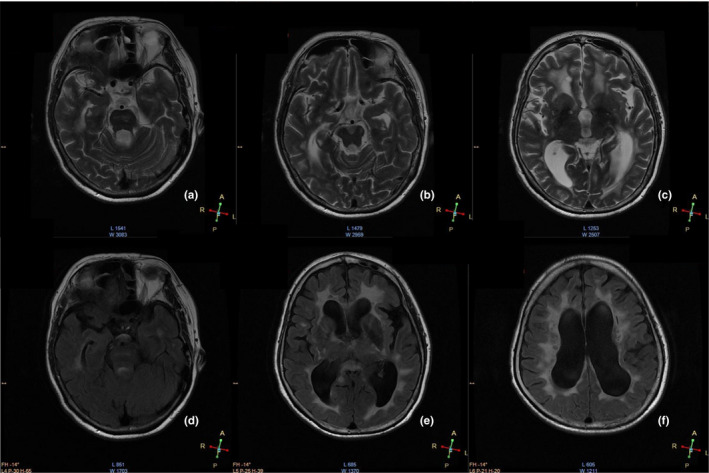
Brain magnetic resonance imaging (MRI) of 60‐year‐old sister of the proband. Brain MRI of proband's sister: White matter hyperintensities in temporal lobe and periventricular region in T2‐weighted MRI (a–c) and fluid‐attenuated inversion recovery MRI (d–f); diffuse cortical and subcortical white matter atrophy and multiple lacunar infarcts in the pons, cerebral peduncle, and basal ganglion are observed
